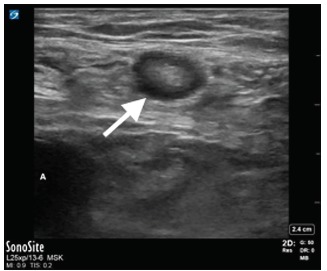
Appendix within the hernia sac. Arrow pointing to the appendix with a diameter of approximately one centimeter. Pressure is being applied with the ultrasound probe, demonstrating that the appendix is non-compressible, while also compressing the femoral vein so it cannot be visualized. The femoral artery can be seen in the lower left (A).
